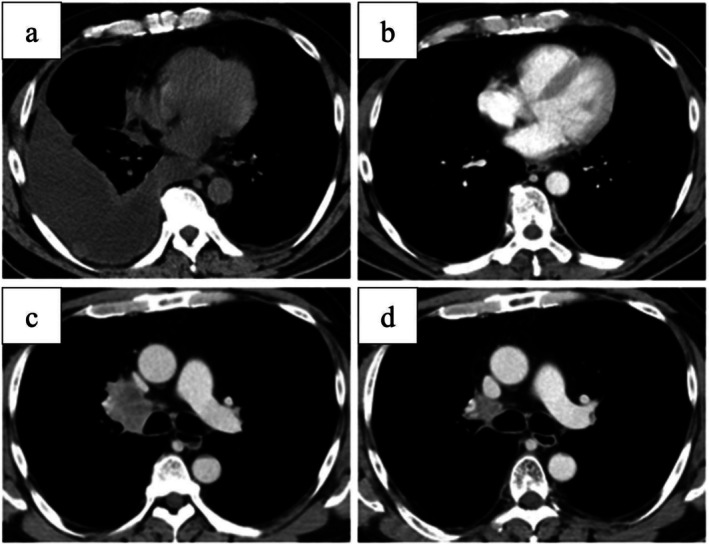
Postoperative imaging findings following gemcitabine and cisplatin chemotherapy for recurrence after radical cystectomy, which proved ineffective (a) Computed tomography (CT) just before initiating pembrolizumab therapy, revealing massive pleural effusion accumulation, suggesting progression to cancerous pleurisy caused by pleural dissemination. (b) CT at 6 months after the start of pembrolizumab: Complete response was achieved. (c, d) CT at 15 months after the beginning of pembrolizumab: an enlarged lymph node appeared in the right hilar region. It was determined to be oligoprogressive, and radiation therapy was administered, resulting in tumor shrinkage.
